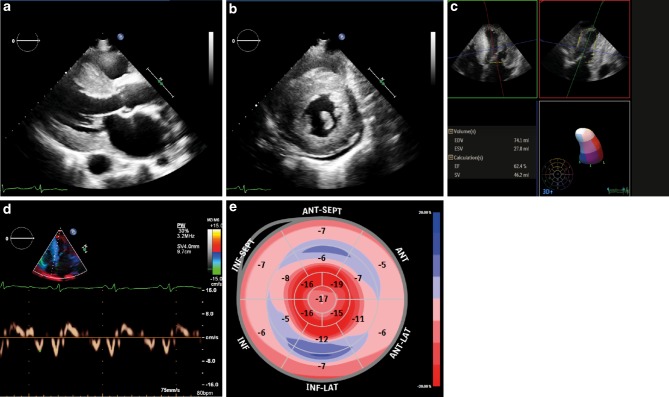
Echocardiography in cardiac amyloidosis. a–b Granular echogenic appearance of the left ventricular wall with clear hypertrophy and some pericardial effusion. Left ventricular ejection fraction is preserved (c), septal tissue Doppler longitudinal movement is reduced (d). Longitudinal strain analysis from the 3 apical views showing characteristic apical sparing (bull’s eye) with reduced strain at the mid and basal level (e)
